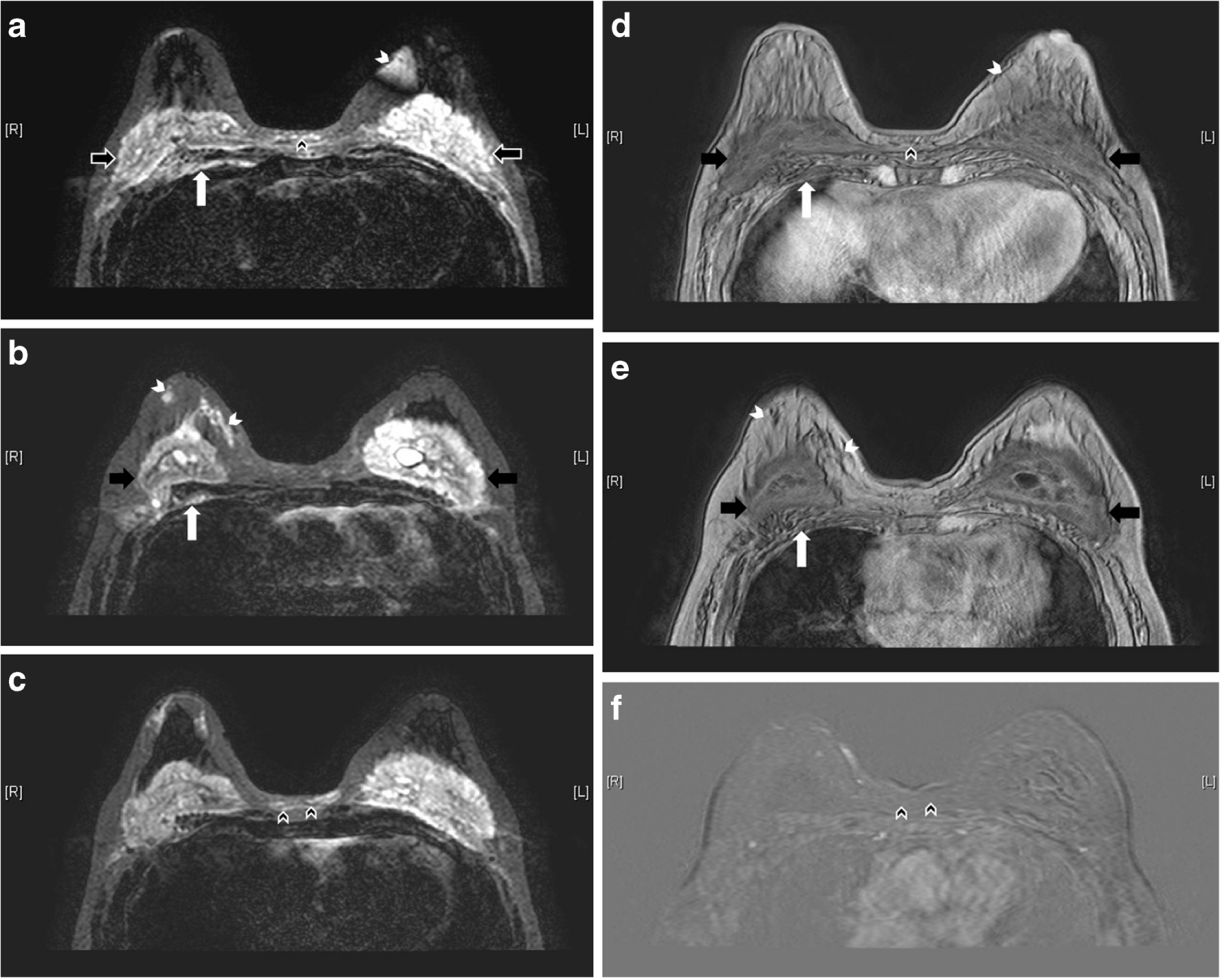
Free silicone injection. High signal collections (black arrows) are noted at the retroglandular regions of both breasts on these axial MR images of silicone-only sequence (a–c), compatible with free silicone. Collections are also seen in premammary fat (white arrowheads), retropectoral region (white arrows), and pre-sternal region (black arrowheads). They are suppressed on silicone-saturated sequence (d, e). No enhancement is noted over the pre-sternal collection (black arrowheads) on T1-weighted post-contrast image with subtraction (f)
